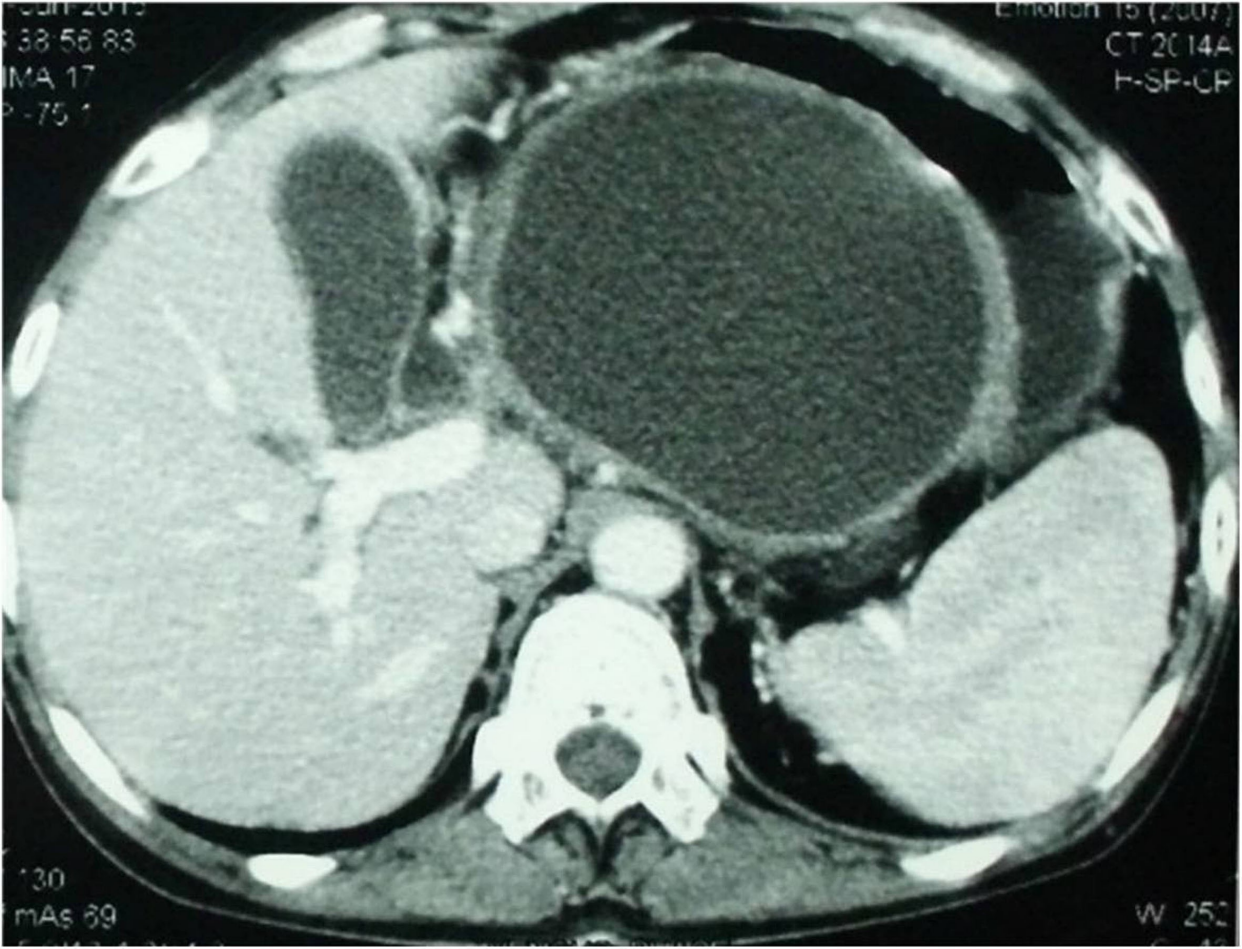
CT scan of the abdomen showing a large pseudocyst in the body and tail of the pancreas
